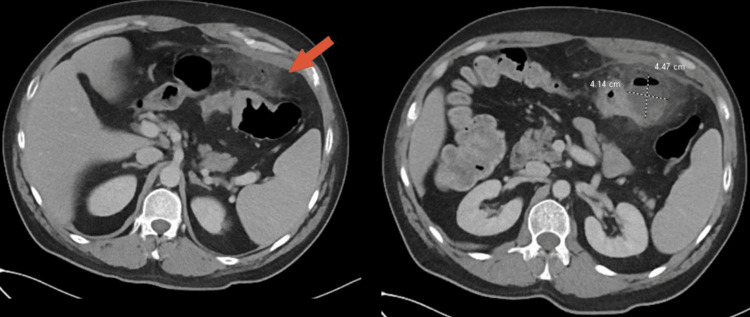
CT abdomen and pelvis with contrast demonstrating evidence of perforated diverticulitis with abscess formation measuring 4.14 x 4.47 x 5.12 cm. Discrete hypodense collection in the left upper quadrant with evidence of stranding and inflammatory changes adjacent to the transverse and splenic flexure (arrow). CT, computerized tomography
